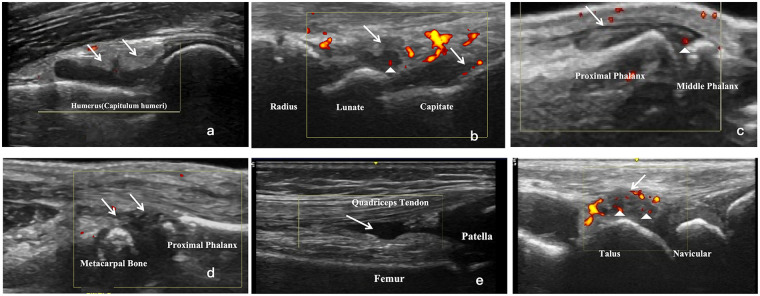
Representative musculoskeletal ultrasound images of key joints included in the subtype-specific simplified assessment models. All images were obtained using high-frequency linear array probes (7–18 MHz) under standardized grayscale (GS) and Power Doppler (PD) settings, in accordance with the pediatric OMERACT scanning protocols. Synovitis severity was graded using a semiquantitative scoring system (GS: 0–3; PD: 0–3). Panels (a–d) show joint sites included in the pJIA simplified model, and panels (e–f) show joint sites included in the oJIA simplified model. White arrows indicate synovial thickening; arrowheads indicate Power Doppler signal reflecting synovial hypervascularity. All machine-embedded text and institutional identifiers were removed prior to submission. (a) Elbow joint, posterior longitudinal scan (pJIA, female, 14 years): marked synovial thickening in the posterior joint recess (arrows), with no detectable Power Doppler signal (GS grade 3, PD grade 0). H, humerus (capitulum humeri). (b) Wrist joint, dorsal longitudinal scan over the radiocarpal and midcarpal compartments (pJIA, female, 10 years): dorsal intercarpal synovial thickening (arrows) with active Power Doppler signal indicating synovial hypervascularity (arrowhead; GS grade 2, PD grade 2). R, radius; Lu, lunate; Ca, capitate. (c) Third proximal interphalangeal (PIP3) joint, dorsal longitudinal scan (pJIA, male, 9 years): synovial thickening of the dorsal joint recess (arrow) with mild Power Doppler signal (arrowhead; GS grade 3, PD grade 1). PP, proximal phalanx; MP, middle phalanx. (d) First metacarpophalangeal (MCP1) joint, dorsal longitudinal scan (oJIA, male, 3 years): marked synovial thickening occupying the dorsal recess (arrows), with no detectable Power Doppler signal (GS grade 3, PD grade 0). MC, metacarpal bone; PP, proximal phalanx. (e) Knee joint, suprapatellar longitudinal scan (oJIA, male, 7 years): synovial thickening and joint effusion within the suprapatellar recess (arrow), with no detectable Power Doppler signal (GS grade 2, PD grade 0). F, femur; QT, quadriceps tendon; P, patella. (f) Ankle joint, anterior longitudinal scan over the tibiotalar joint (oJIA, male, 7 years): synovial thickening (arrow) with prominent Power Doppler signal (arrowheads; GS grade 3, PD grade 2). Ta, talus; Na, navicular. GS, grayscale; PD, Power Doppler; pJIA, polyarticular juvenile idiopathic arthritis; oJIA, oligoarticular juvenile idiopathic arthritis; PIP, proximal interphalangeal; MCP, metacarpophalangeal.
